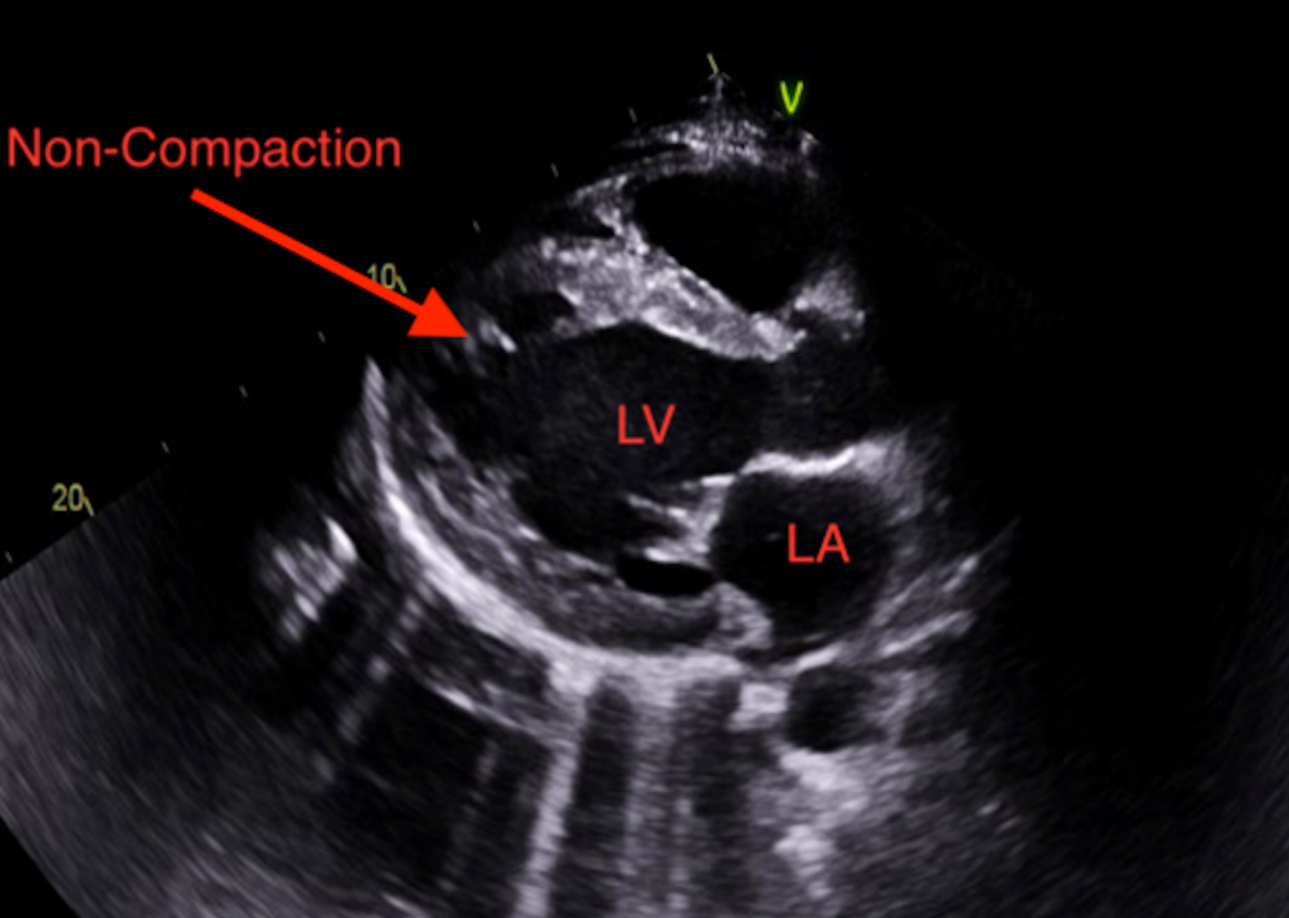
Parasternal long axis view showing LVNC (left ventricular non-compaction). LV: Left ventricle; LA: Left atrium.
